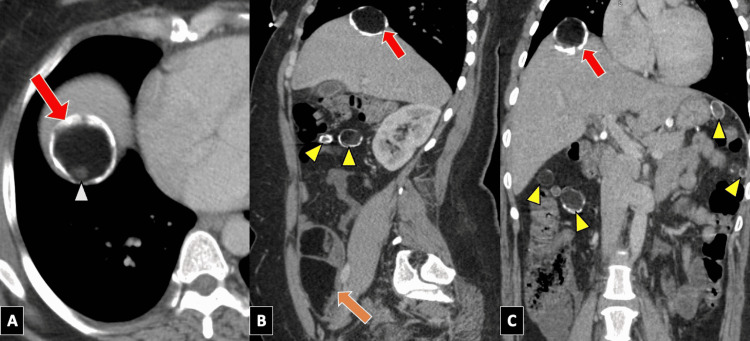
Axial (A), sagittal (B) and coronal (C) contrast-enhanced abdominal CT demonstrating a complex adnexal mass with fat and soft tissue attenuation (orange arrow). A partly calcified mixed attenuation mass is also seen at the dome of the liver (red arrows), representing one of many peritoneal deposits from a ruptured ovarian dermoid. This mass corresponds to the lesion seen on the initial ultrasound. A rounded soft tissue component within this lesion represents a characteristic Rokitansky nodule (white arrowhead). Multiple additional peritoneal and mesenteric dermoid implants are also seen (yellow arrowheads). CT: computed tomography.
